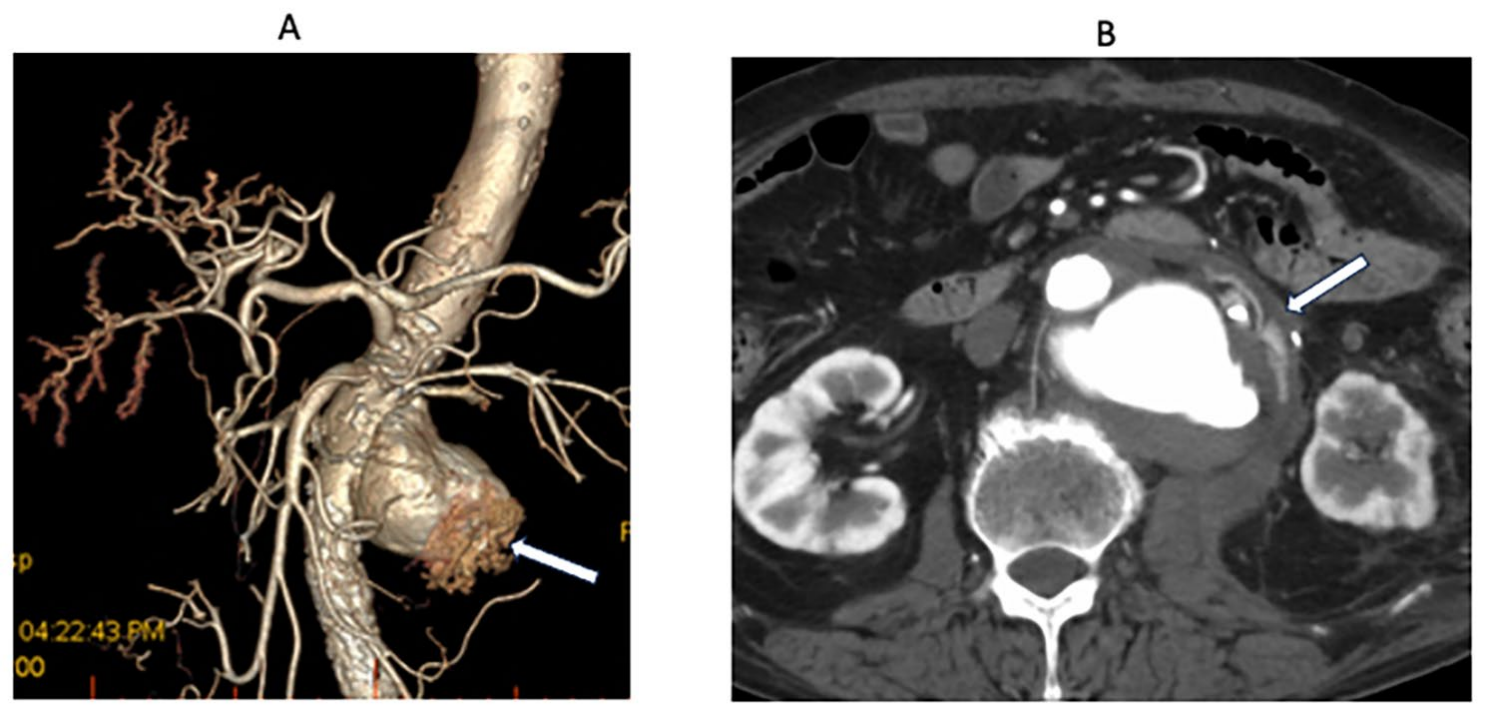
A and B: Computed tomography (CT) (day 12) after the initial operation shows the patch closure of the ruptured site (white arrow)
